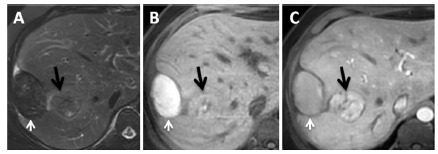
MRI in a patient with adenoma with bleeding: A) a T2 sequence with fat saturation shows a hepatic subcapsular nodule with hyposignal; B) a pre-contrast T1 sequence reveals a lesion with hypersignal, indicating products of hemoglobin degradation; C) a post-contrast T1 sequence in the arterial phase emphasizes the intra-hepatic lesion where the black arrow points to the lesion and the white arrow to the hematoma.
